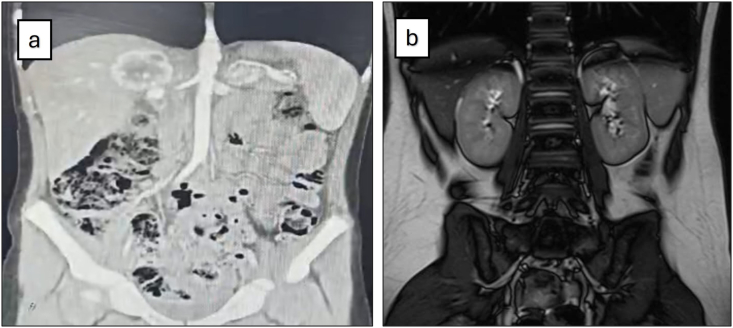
(a) CT scan revealed a hyperechoic mass measuring 52 × 45 mm in the anatomical position of the right adrenal gland and a similar but smaller solid lesion measuring 29 × 20 mm in the left adrenal gland. (b) Follow up MRI showed no residual masses.
